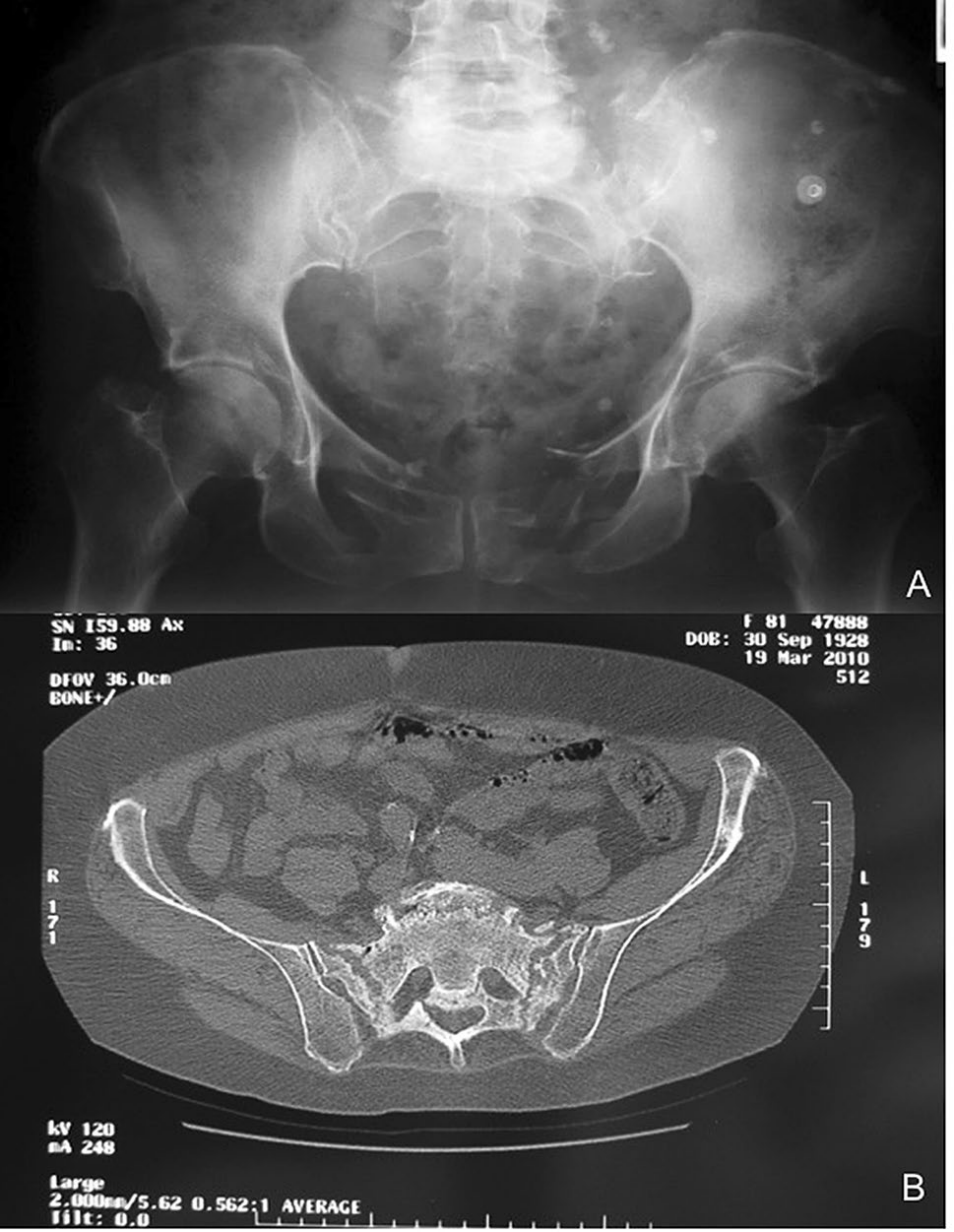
Preoperative X-ray and CT scan
